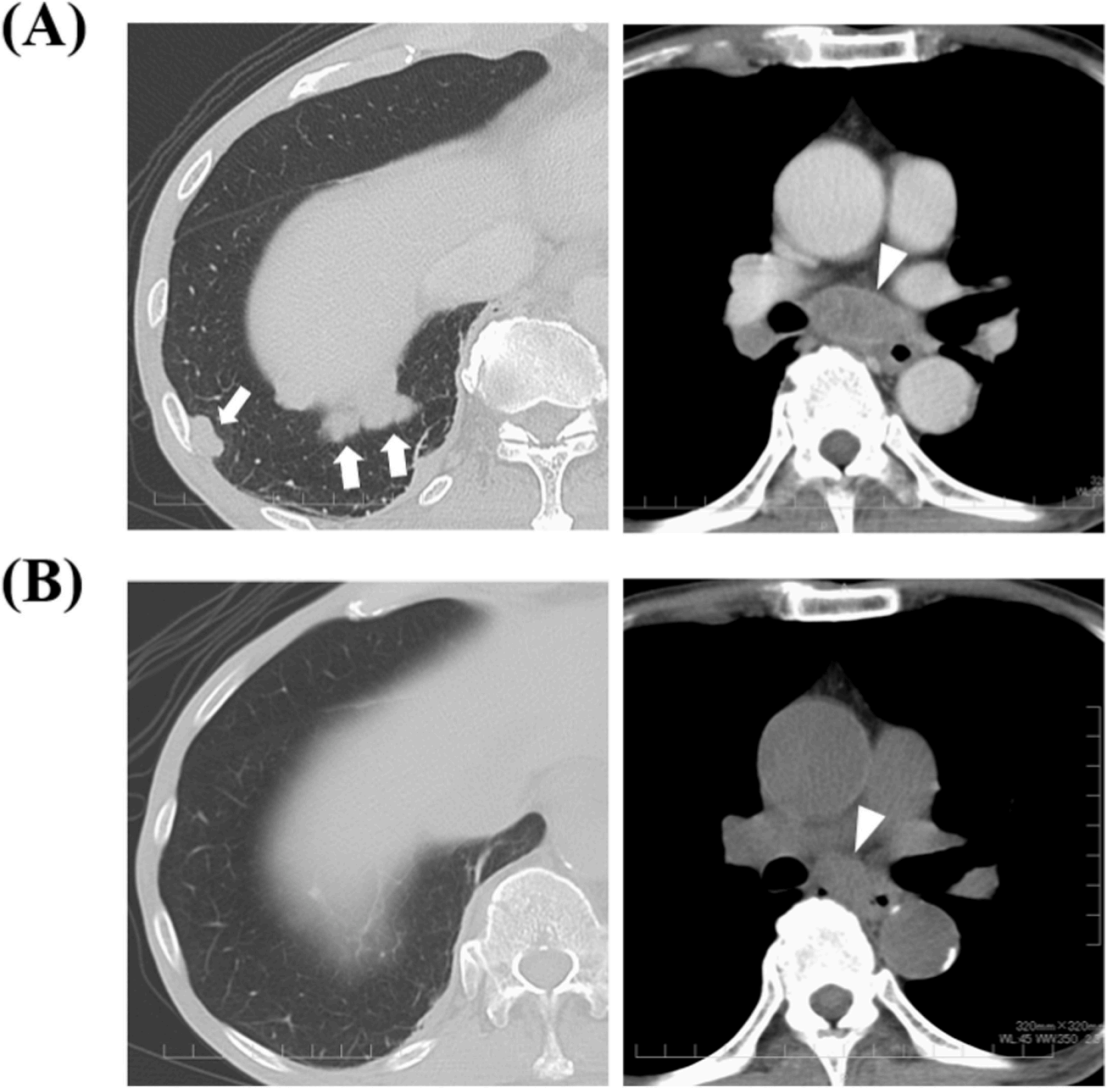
Chest CT at diagnosis (A) and 20 days after starting of chemotherapy (B). A: Chest CT at diagnosis showed multiple nodules in the right lower lobe (*arrows*) and subcarinal lymphadenopathy (*arrowhead*). B: Chest CT at 20 days after starting chemotherapy. Both the lung nodules and the lymph node metastasis were reduced in size (*arrowhead*).
